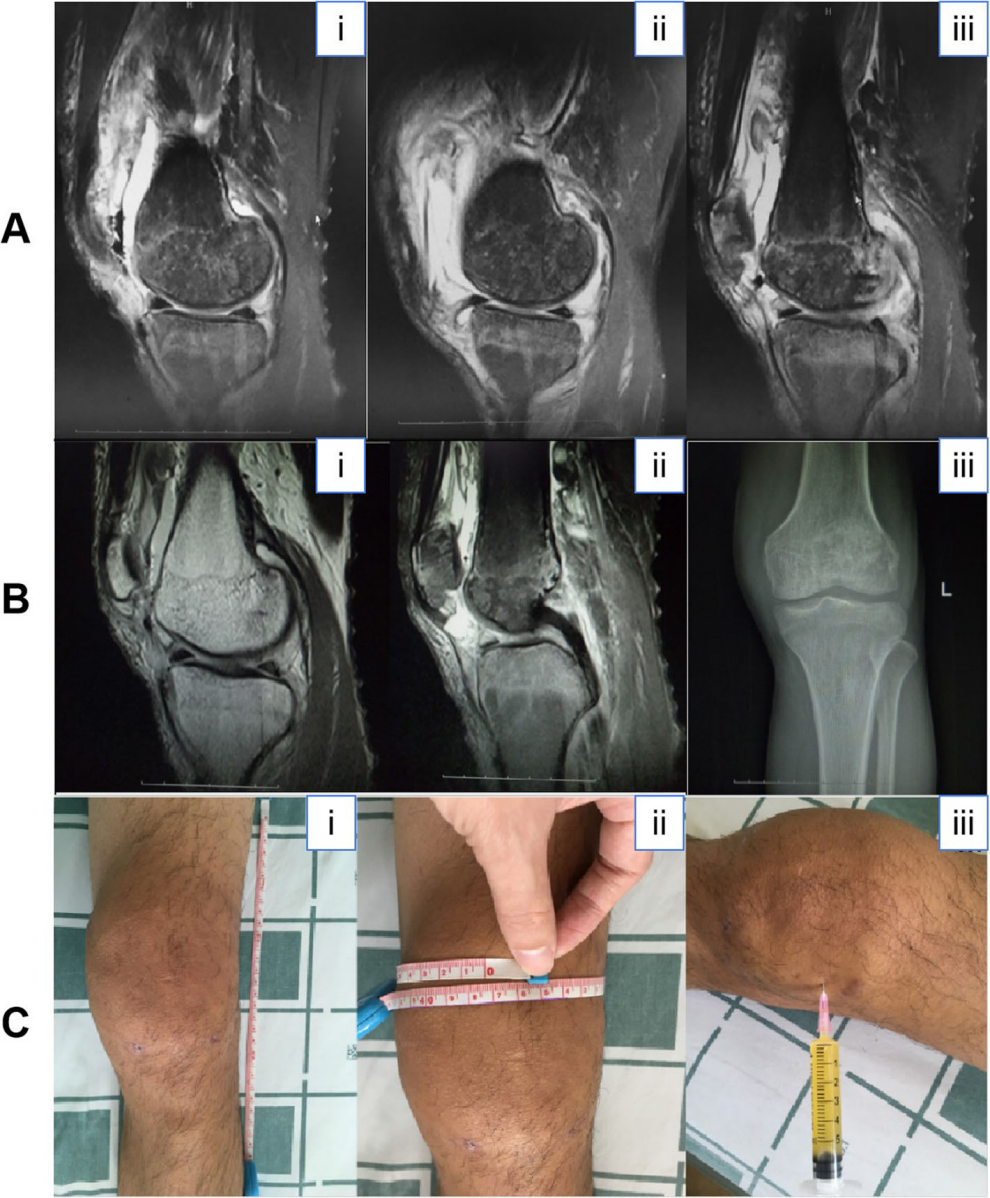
a. MR imaging indicated bone marrow edema of the proximal tibia, distal femur and patella, posterior horn tears of the medial and lateral meniscus (III°), and joint effusion, all of which confirmed the clinical diagnosis of purulent arthritis. b(i,ii). MR imaging after knee arthroscopic surgery revealed that the joint swelling was reduced, the proximal tibia edema was improved, and the left lateral meniscus was repaired, while the other conditions were similar to those observed in the first MR images. b(iii). Left knee DR revealed swelling of the soft tissue of the left knee joint, and the bone was loose, indicative of osteoporosis. c. The left knee was swollen; the longitudinal range of swelling was 29 cm, and the knee circumference at the most swollen part was 47.4 cm. Purulent fluid was withdrawn from the left knee
